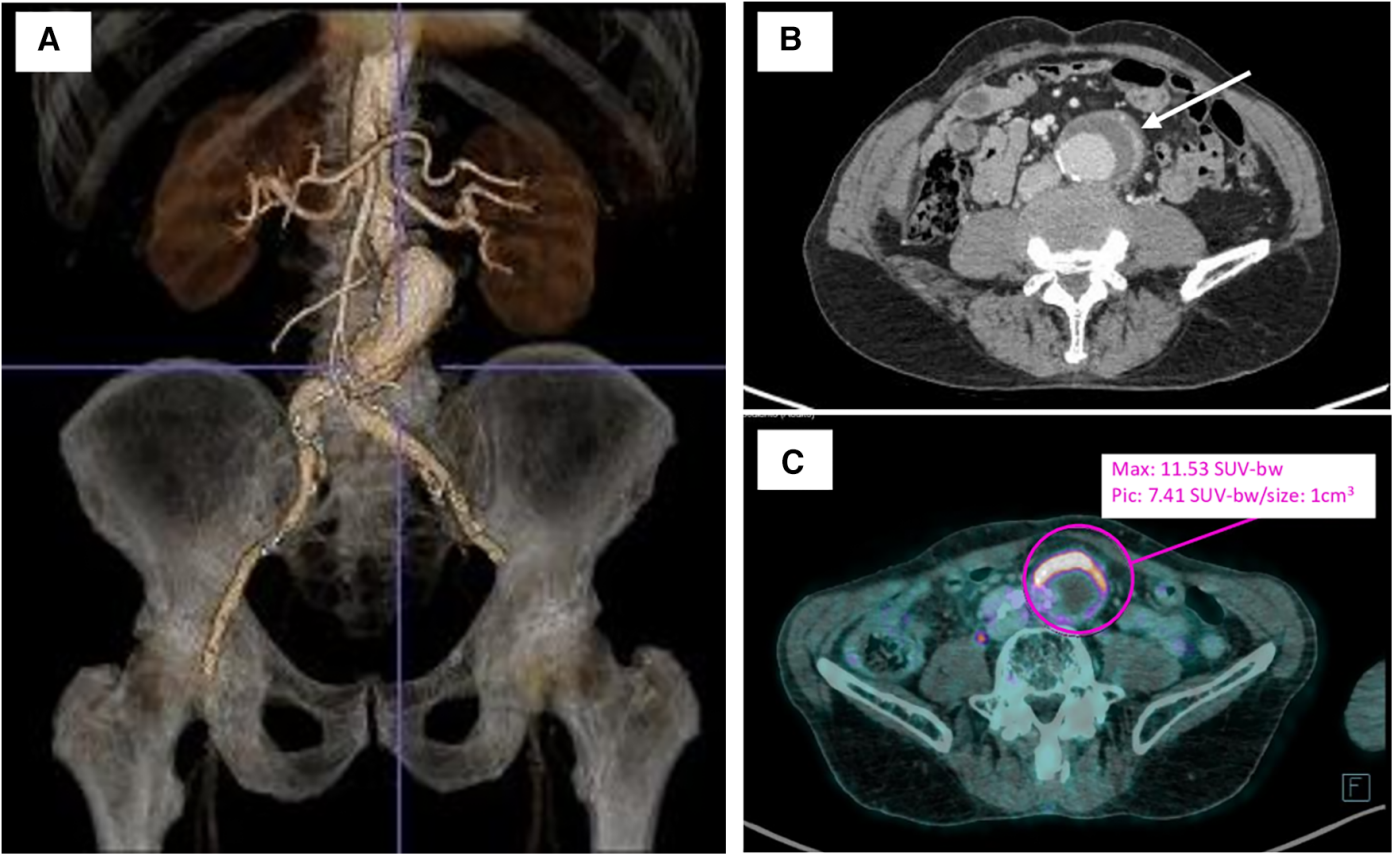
Preoperative imagery of infrarenal aortic aneurysm. (A) Three-dimensional computed tomography aortic aneurysm reconstruction with the TeraRecon Aquarius workstation preoperatively. (B) Preoperative computer tomography angiography of the infected abdominal aortic aneurysm with anterior aortic wall thickening and enhancement (white arrow). (C) Preoperative 18FDG-PET/CT revealing a significant uptake of anterior aneurysm wall (purple circle). bw, body weight.
